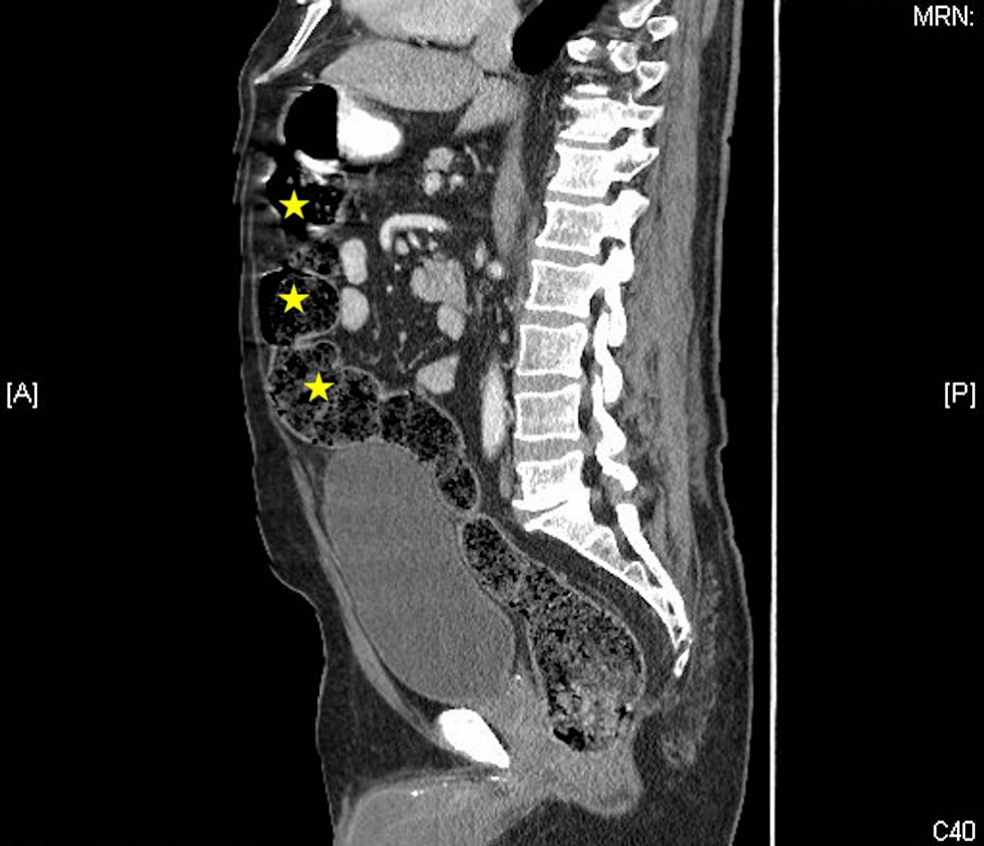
CT scan, sagittal view, demonstrating inflammatory changes surrounding cecal diverticula, consistent with diverticulitis (stars)
